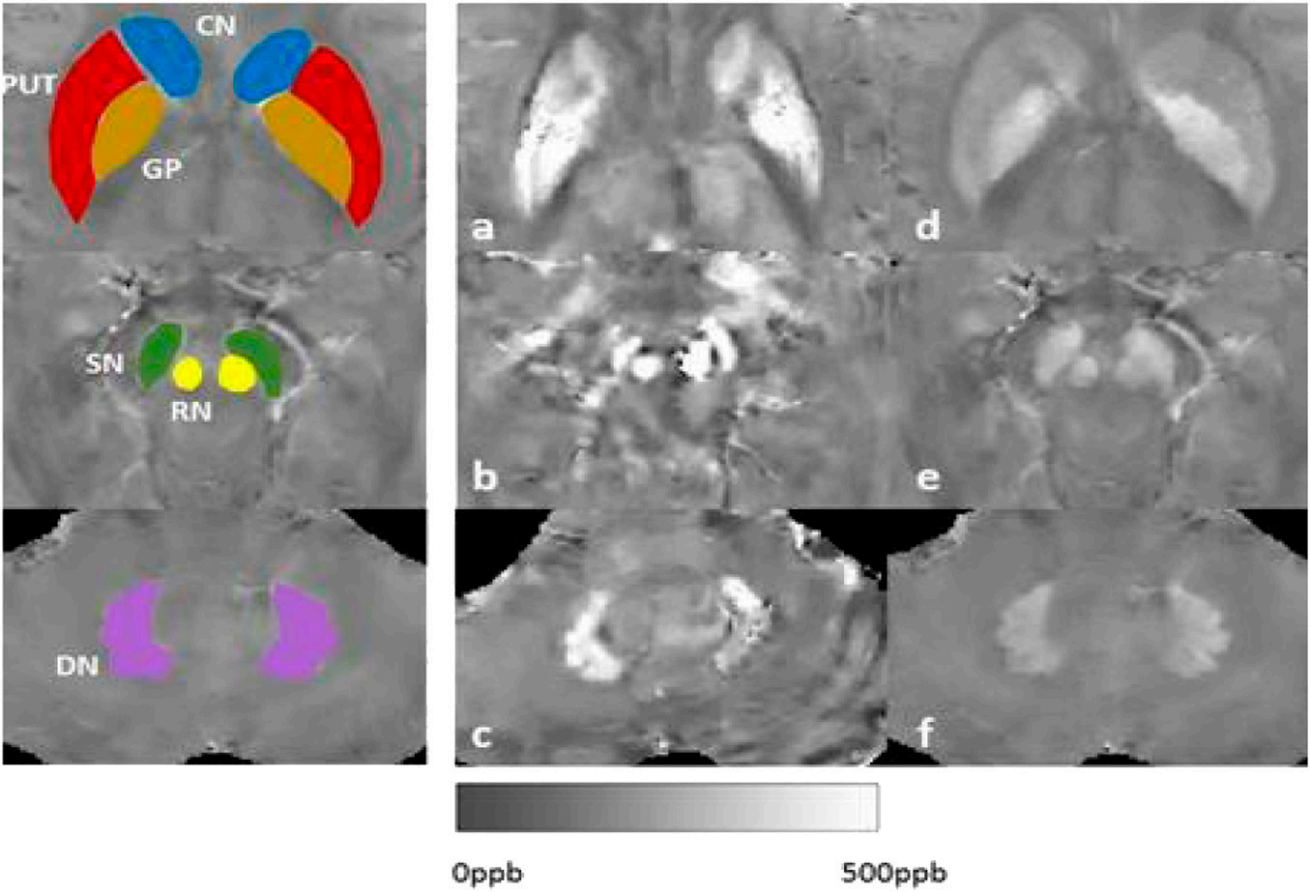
Our results of MRI-QSM signals: iron/copper related Magnetic Susceptibility in the Deep Gray Matter Nuclei of Wilson’s Disease. Note: CN: caudate nucleus; GP: globus pallidus; PUT: putamen; SN: substantia; RN:rednucleus; DN: dental nucleus.
